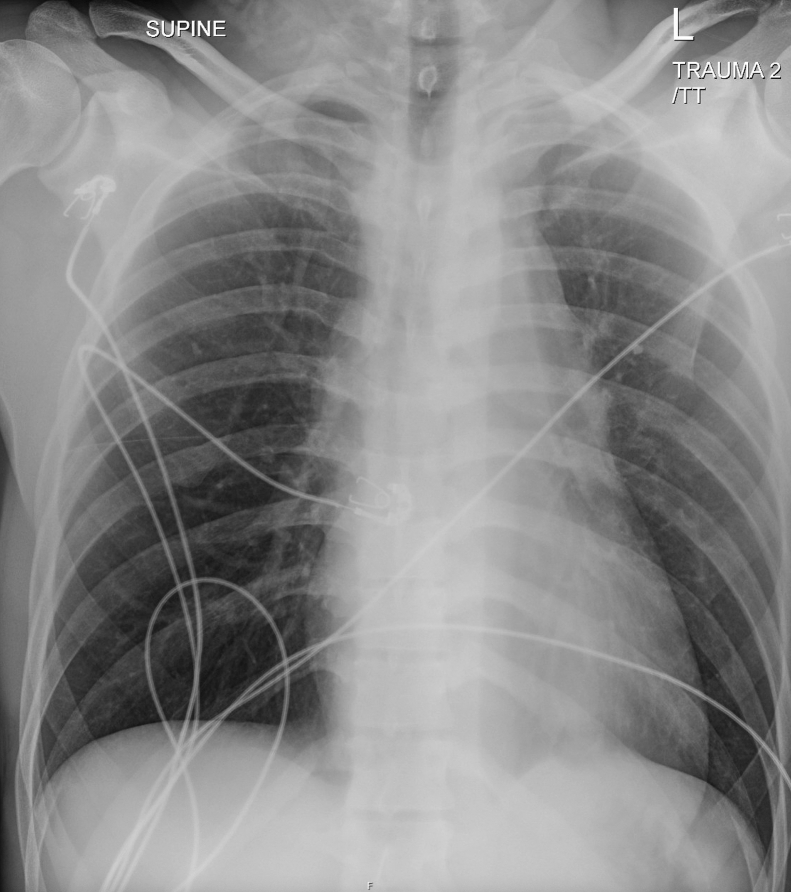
Chest radiograph demonstrating mediastinal widening and loss of the aortic knob.
